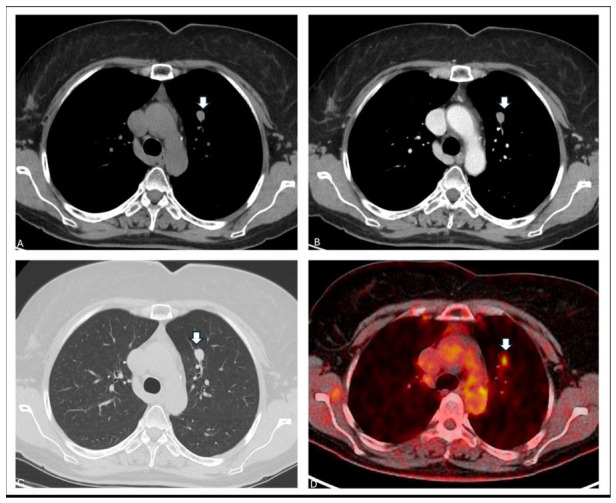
Pulmonary GCT is rare and has historically been estimated to account for approximately 6–10% of all GCTs. Within the respiratory tract, it most frequently arises in the tracheobronchial tree rather than in the peripheral lung parenchyma [1,2]. Endobronchial lesions typically present with symptoms related to airway obstruction, including recurrent pneumonia, hemoptysis, or persistent atelectasis [3]. Large clinical series have shown that a substantial proportion of tracheobronchial GCTs are discovered incidentally and that coexistence with other malignancies is not uncommon [4,5]. Radiologically, pulmonary GCT lacks specific features and may present as a well-defined nodule or endobronchial mass; in oncological patients, as in our case (Figure 1), this appearance may raise concern for metastases. In particular, metastases from papillary renal cell carcinoma usually appear as round lesions with smooth margins and their FDG uptake can be variable [6]. Similarly, FDG uptake in granular cell tumor is variable and may overlap with both benign and malignant lesions [7]. Advanced imaging techniques like radiomics, favoring the distinction between benign and malignant nodules—including metastases—might be applied in the future in similar circumstances providing additional information [8,9]. In the present case, a contrast-enhanced chest computed tomography (CT) was performed during routine oncologic follow-up for papillary renal cell carcinoma in a 56-year-old woman, which revealed a solid peribronchovascular pulmonary nodule in the anterior segment of the left upper lobe. The chest CT nicely showed the 12 mm nodule with smooth margins and homogeneous contrast enhancement in the left upper lobe (white arrows) ((A): axial mediastinal window with contrast; (B): axial mediastinal window without contrast; (C): lung window). The nodule did not show any contact with the pleura, cavitation or calcification. The axial fused [^18^F] FDG/PETCT (Siemens Biograph mCT 20 Excel; no further technical information was available due to the retrospective study design) showed mild tracer uptake (SUVmax 4 and SUVmean 1.4) of the nodule (white arrow) (D).
